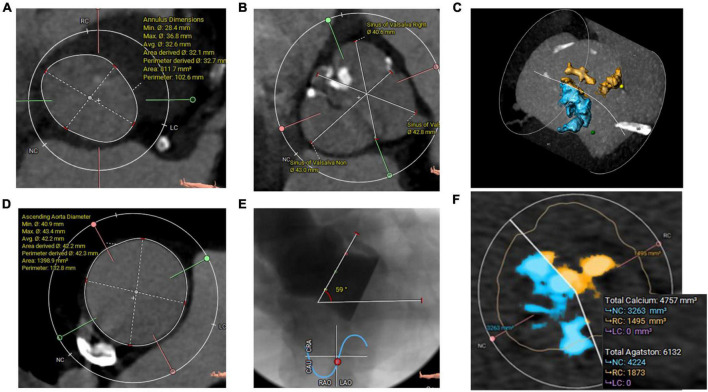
(A) CCTA Annulus measurements with a very large area, (B) wide sinuses, (C) all calcifications (3D Hockey Puck-MIP view), (D) ascending aorta diameters, (E) angiographic aortic angulation showed horizontal aorta, and (F) aortic valve calcifications (Agatston and volume mm^3^) measured from non-contrast CT scan.
